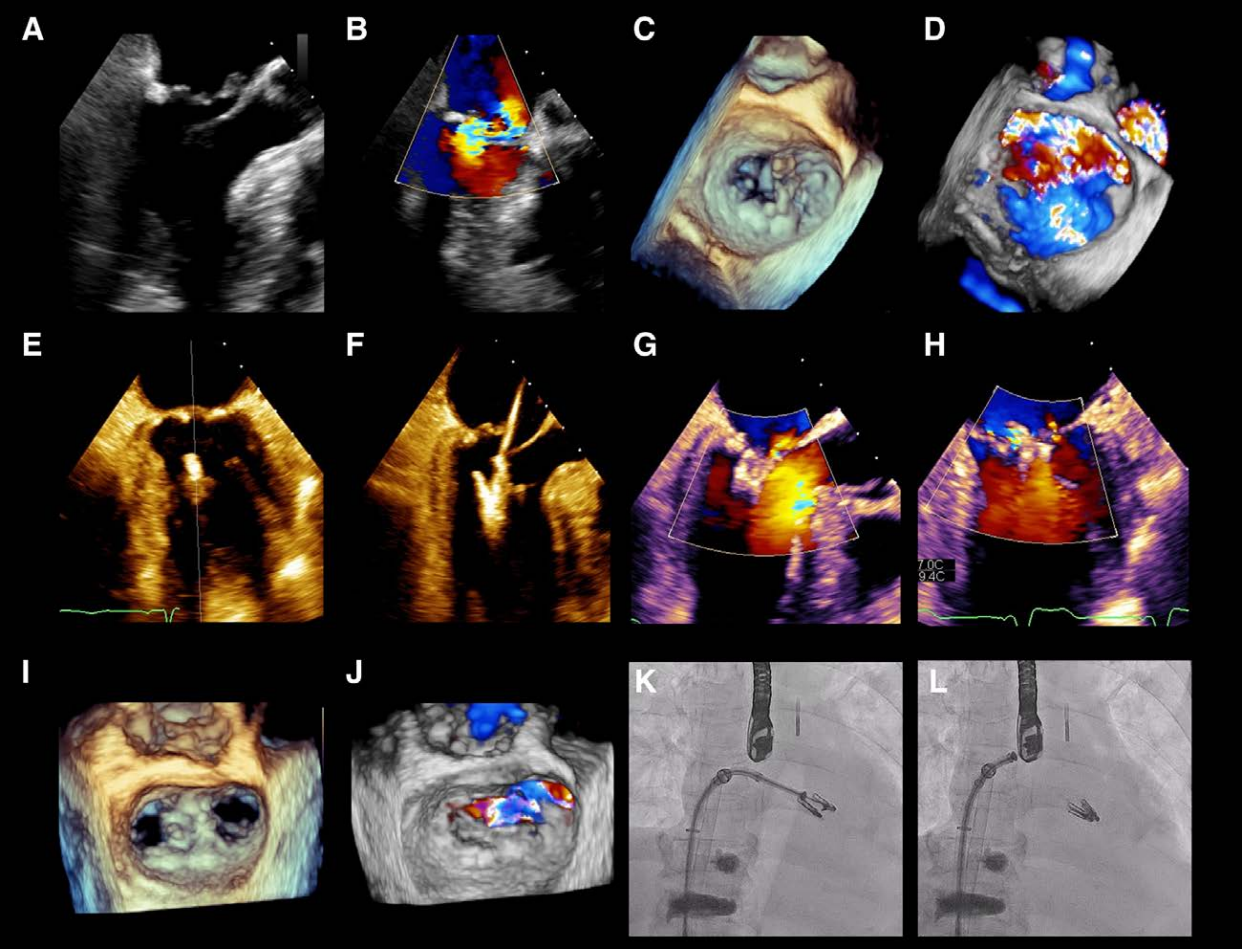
Echocardiography and fluoroscopic images of transcatheter edge-to-edge repair. (A and B) Transesophageal echocardiography (TEE): mid-esophageal long-axis view and color flow depicting severe eccentric mitral regurgitation (MR) due to prolapsed posterior leaflet. (C and D) 3D-TEE image and color flow demonstrating ruptured posteromedial papillary muscle and associated posterior (P2 scallop) leaflet flail with huge MR. (E and F) X-plane view demonstrating implantation of the first clip. (G and H) X-plane view with color flow demonstrating mild MR after implantation of 2 clips. (I and J) 3D-TEE image with surgical *en face* view of mitral valve depicting double orifice after implantation of 2 clips and mild MR. (K and L) Fluoroscopic image after implantation of 2 clips. 3D-TEE = three-dimensional transesophageal echocardiography.
